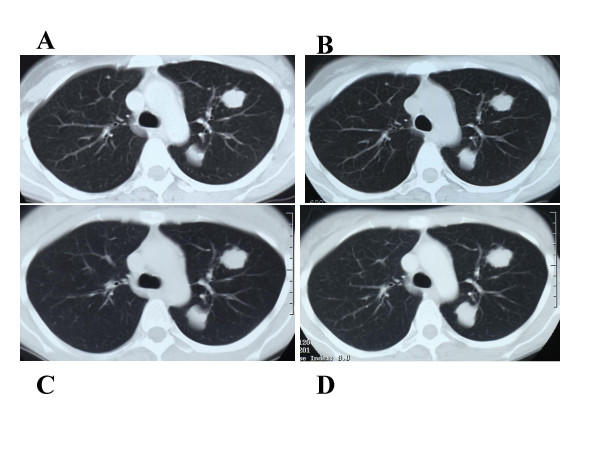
CT scan image of the lung of case 5 patient. A: Before vaccination (July 8, 2003). B: After the first vaccination (July 22, 2003). C: After the third vaccination (August 19, 2003). D: After the sixth vaccination (September 16, 2003). The metastatic tumors appeared to be dormant after the first vaccination.
